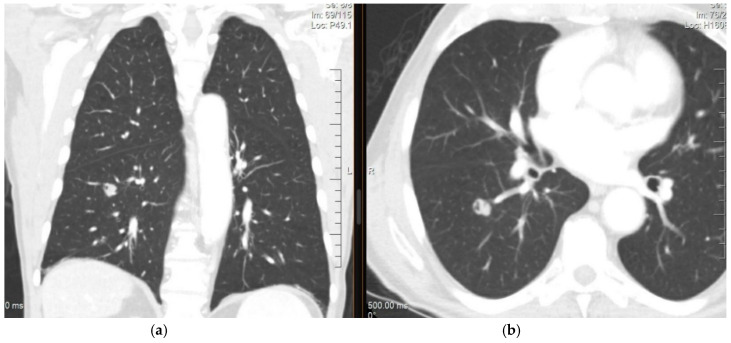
CT of the chest (a) coronal view and (b) axial view showing a nodule in the right lower lobe measuring 1.5 cm, lobulated and cavitated. The lesion was biopsied and revealed an adenocarcinoma with cytomorphology and an immunoprofile that was consistent with a colorectal primary.
